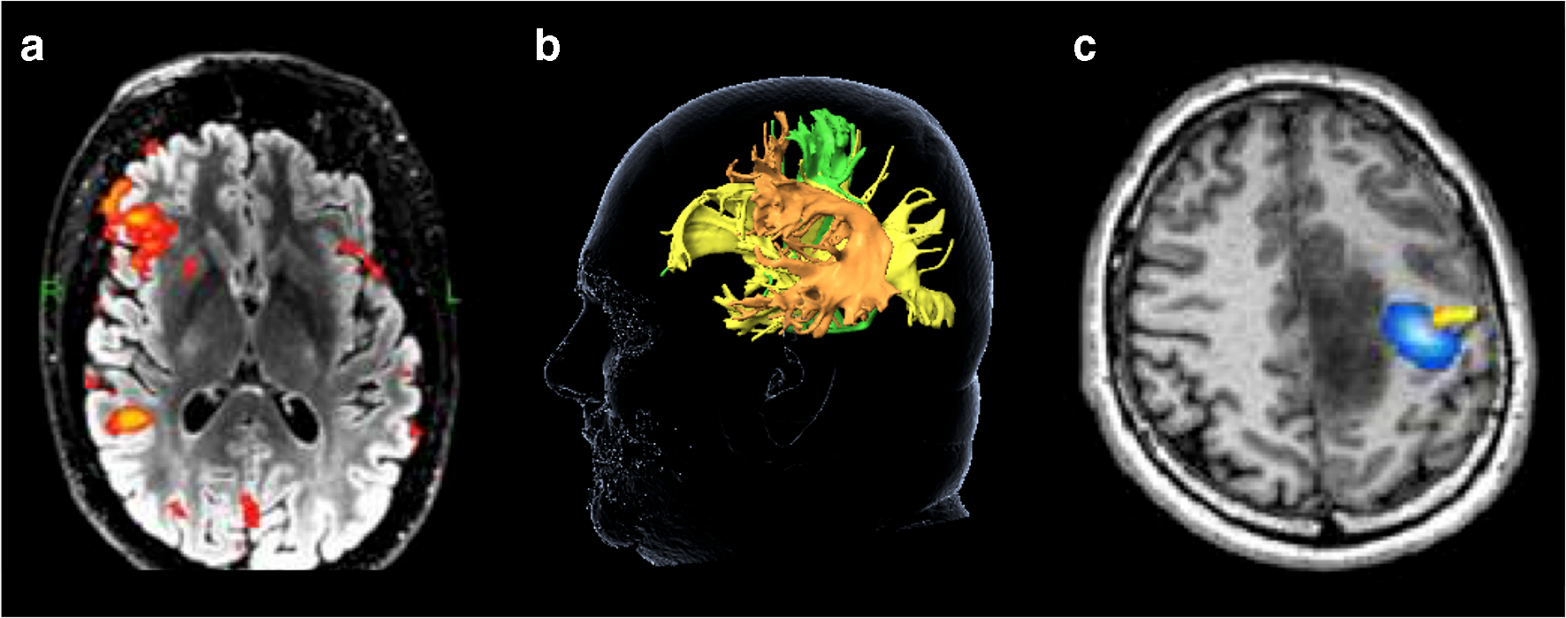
Examples of imaging for localization of brain functions and white matter tracts. A Functional MRI of a patient with a language located in the right hemisphere, which was confirmed with a WADA test. B Visualization of the left corticospinal tract (green), fasciculate arcuatus (orange) and inferior fronto-occipital fasciculus (yellow) using DTI in a patient with a left temporal diffuse astrocytoma, IDH-mutant. C Localization of the left motor cortex using MEG in a patient with a diffuse astrocytoma, IDH-mutant
